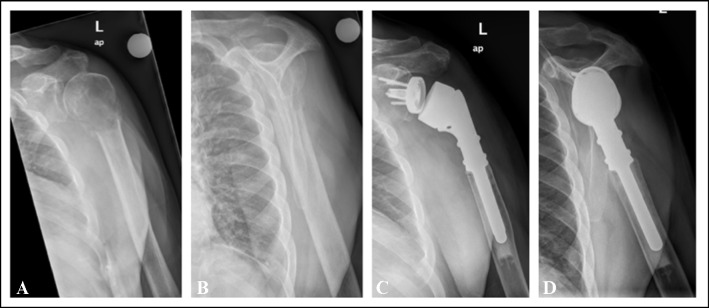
Singular bone metastasis of renal cell carcinoma in a 68-year-old patient, presenting as the primary clinical manifestation of the cancer initially treated as simple shoulder pain. A, B Radiograph images (anteroposterior and lateral views) of the shoulder and humerus demonstrate a pathological fracture through a subcapital osteolytic lesion. C, D Postoperative radiograph images (anteroposterior and lateral views) of the shoulder and humerus following reverse proximal humerus replacement after curative intraarticular resection
